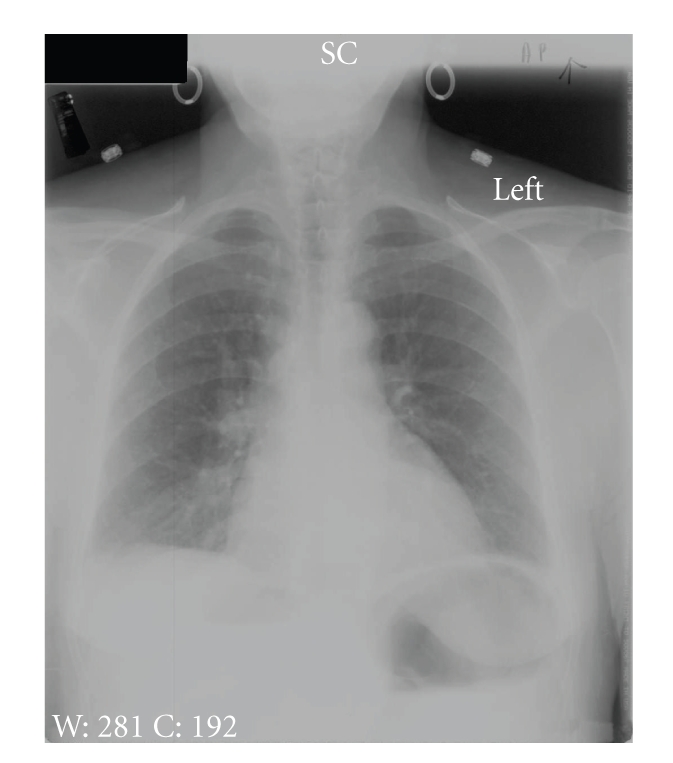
Patient's initial AP chest radiograph. Increased markings are noted in the right lower lobe, along with a possible small right pleural effusion.
